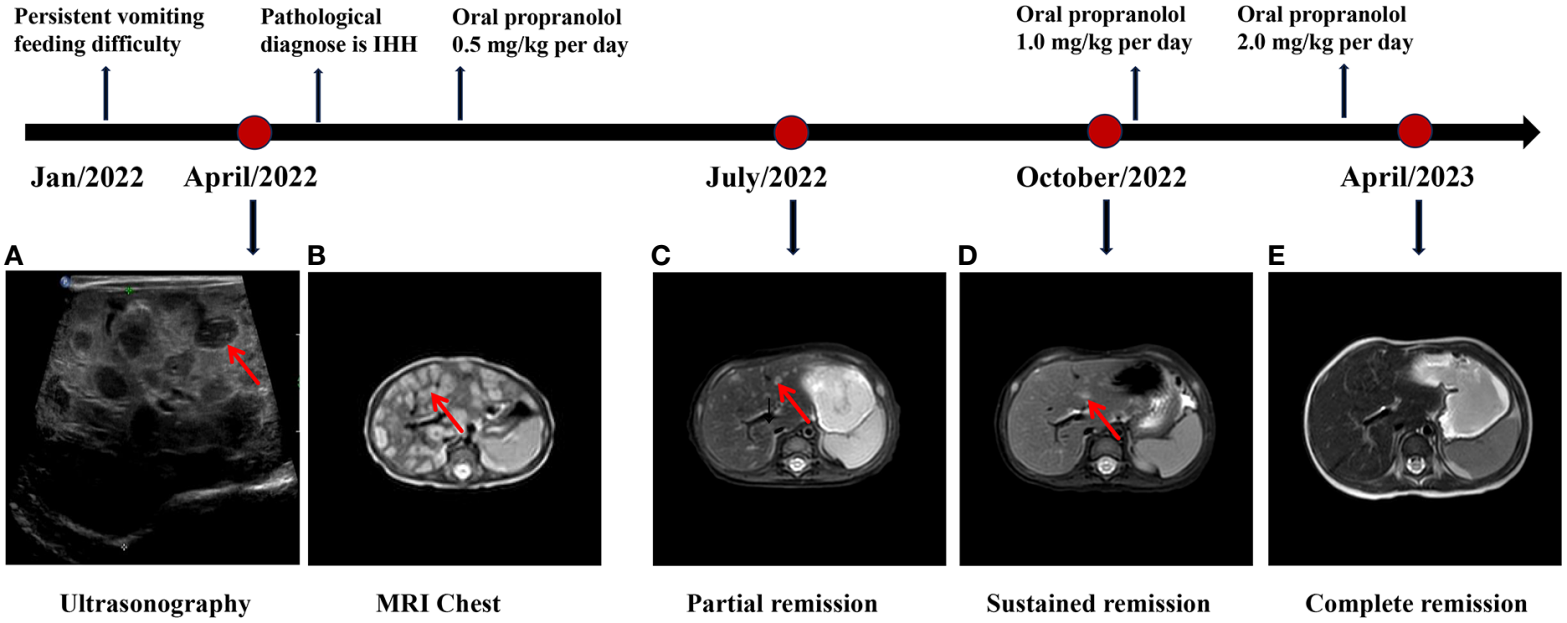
The diagnosis and treatment process of DIHH. (A) The abdominal ultrasonography indicated diffusely heterogeneous hepatomegaly with diffused rounded hypoechoic lesions. (B) The MRI revealed diffuse lesions with hyperintense pattern on the T2-weighted images in the liver. (C-E) The MRI indicates a continuous regression in the lesions during the 6 months follow-up, and ultimately reaching complete remission (CR).
